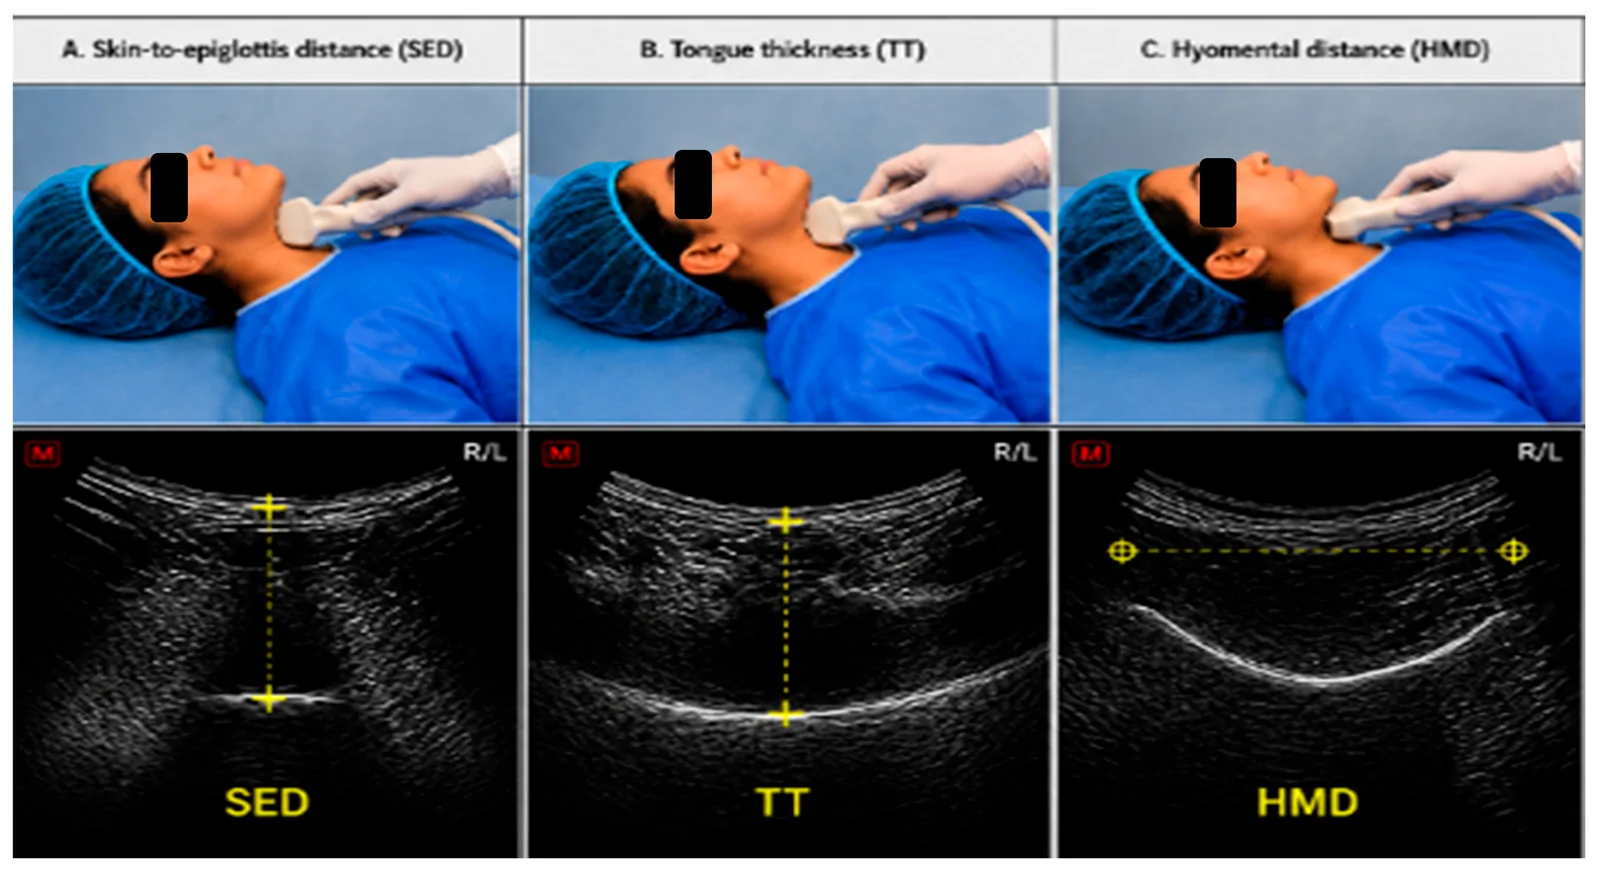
Positioning of the ultrasound probe and corresponding sonographic images used for measurement of (A) skin-to-epiglottis distance (SED), (B) tongue thickness (TT), and (C) hyomental distance (HMD). The yellow calipers and measurement lines indicate the anatomical distances measured for each ultrasonographic parameter. Clinical photographs and ultrasonographic images were obtained by the authors. Probe positioning and measurement techniques were prepared according to the methodology described by Zheng et al. [1].
